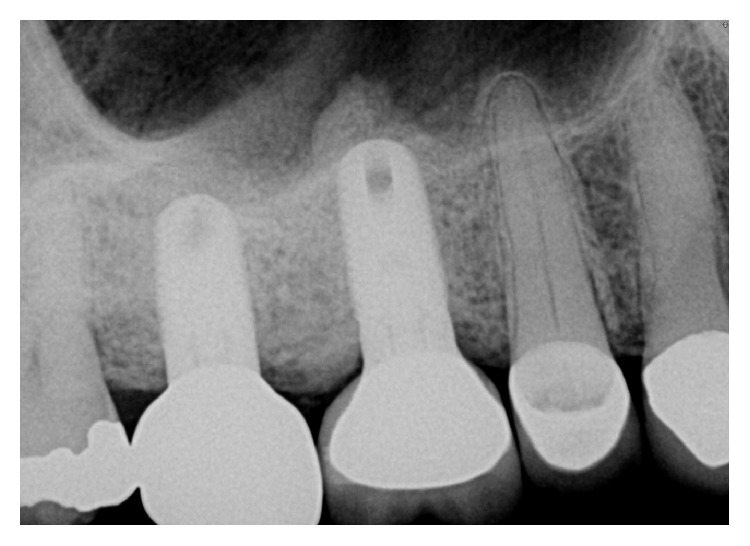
Radiograph at 13 months postoperatively presented increased bone density around the implant apex.
